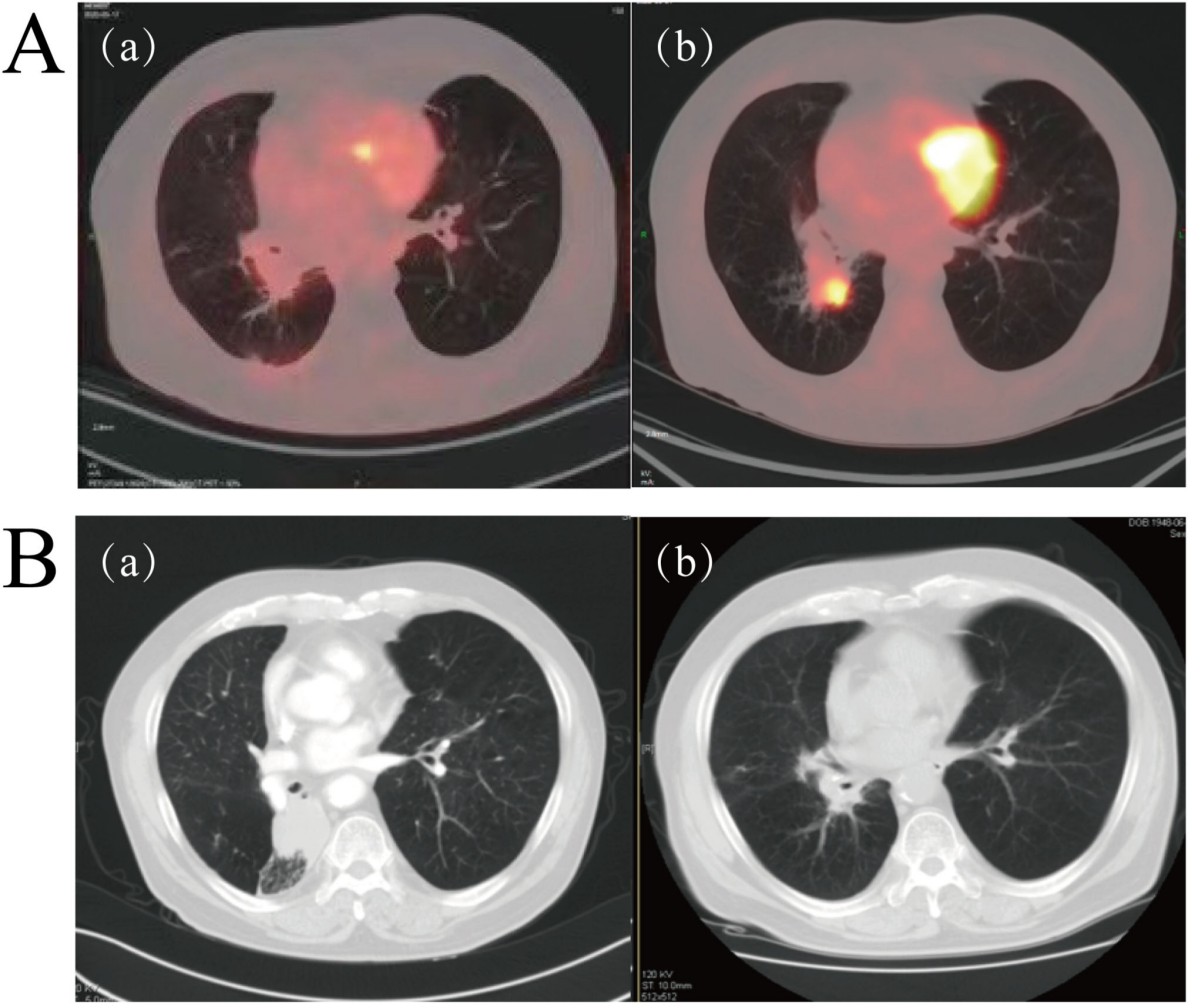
(A) Representative PET/CT imaging during pembrolizumab therapy. (a) PET/CT performed on April 7, 2021, showed a marked reduction in the size of the right lower lobe lesion compared to baseline (Figure 1A). (b) PET/CT conducted on March 31, 2022, demonstrated significant progression of the lesion in the right lower lobe relative to image (A, B) Serial contrast-enhanced chest CT scans before and after radiotherapy. (a) CT scan obtained on November 21, 2022, prior to radiotherapy. (b) CT scan performed on February 20, 2023, approximately six weeks post-radiotherapy completion, indicating complete response (CR).
